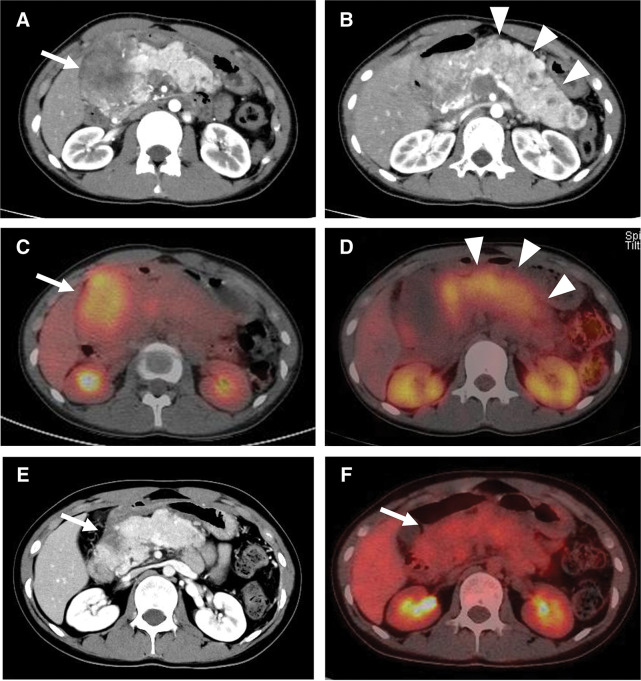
(A) CT shows a hypo-enhanced tumor in the pancreatic head (arrow). (B) CT demonstrates an enhanced tumor in the body and tail in the pancreas (arrowheads). (C) PET/CT shows intense accumulation in the pancreas head tumor (arrow), but not in the body and tail of the pancreas. (D) Somatostatin receptor scintigraphy shows negative accumulation in the pancreatic head mass, but significant accumulation in the body and tail of the pancreas (arrowheads). (E) CT image showing remarkable tumor shrinkage in the pancreatic head after chemotherapy (arrow). (F) PET/CT shows no accumulation in the pancreatic head tumor after chemotherapy (arrow).
